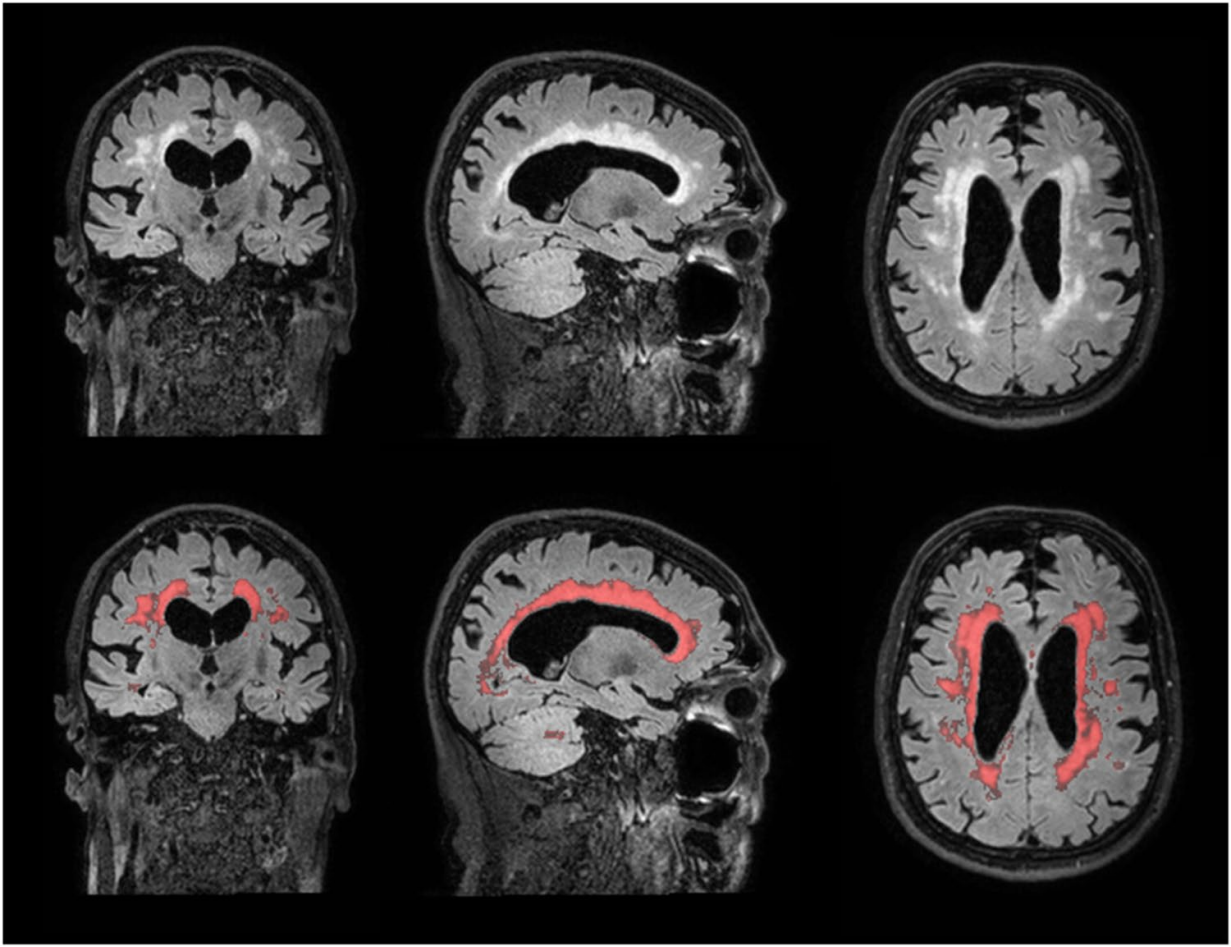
Example of a participant with a high WMH volume and complex WMH shape (left: original 3D FLAIR image; right: FLAIR image with overlay of the segmented WMH probability map in red).
